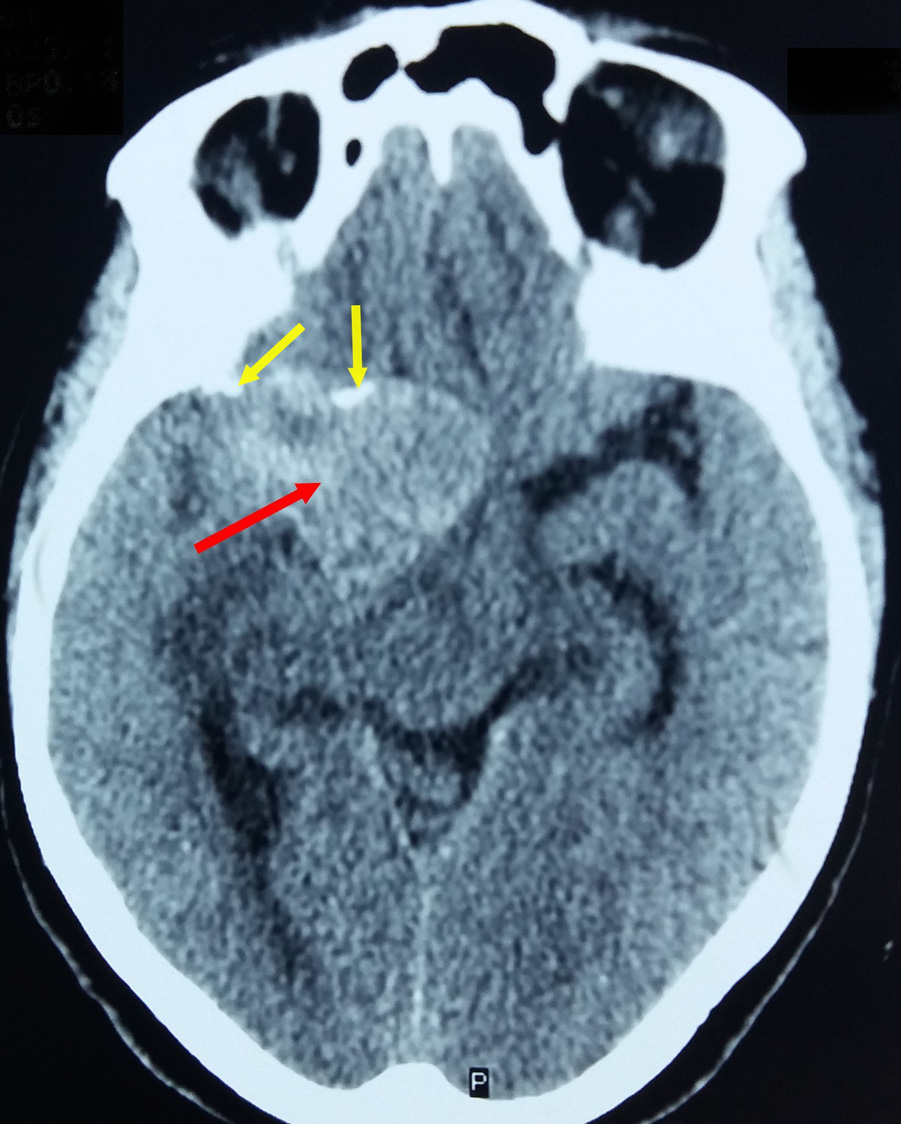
Axial non–enhanced computed tomography (CT) scan in parenchymal window showing a right extraaxial anterior temporal parasellar lesion (red arrow) probably originating from the anterior clinoid process with cranial extension holding calcifications (yellow arrows). The whole was surrounded by perilesional edema resulting in a subfalcine herniation and mass effect on the brainstem (Color version of the figure is available online.)
